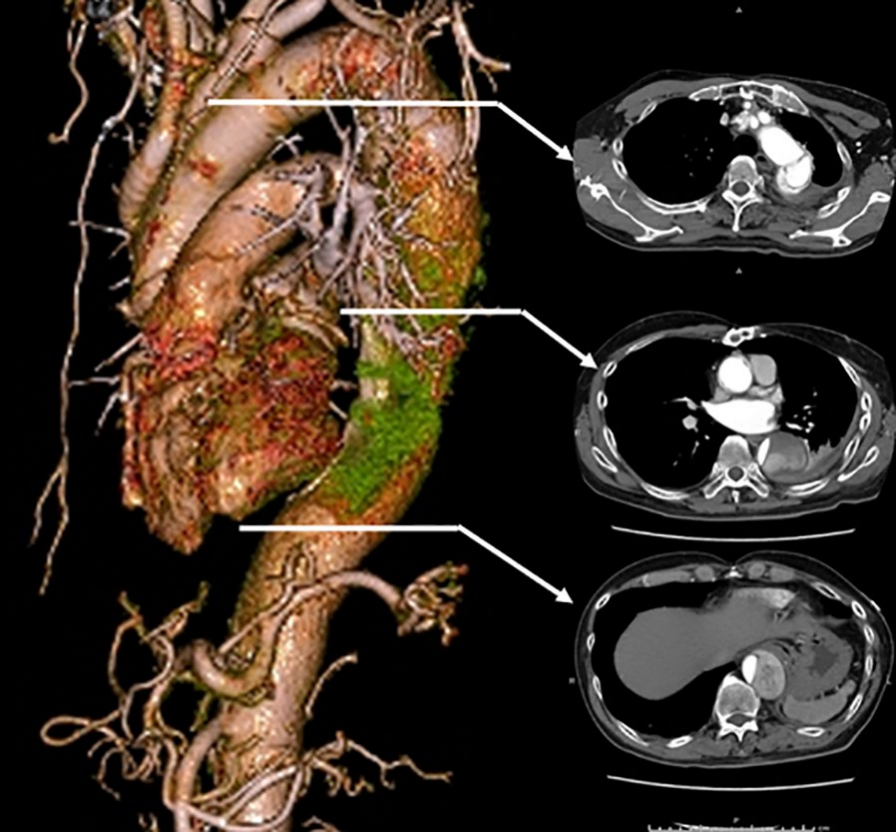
Enhanced computed tomography imaging. A preoperative CT scan showed aneurysmal dilatation of the mid descending thoracic aorta with a maximum diameter of 54 mm
